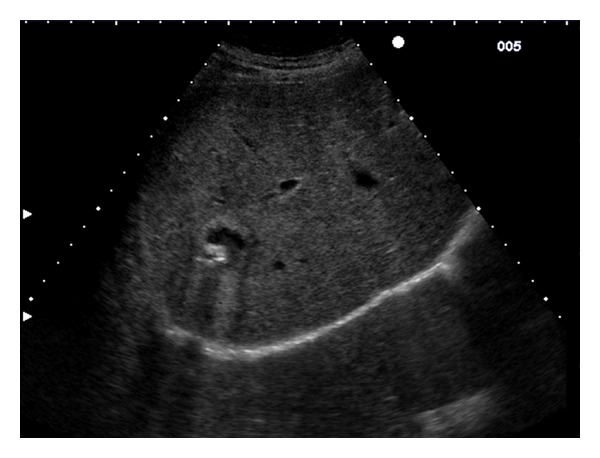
Ultrasound appearance of a brucellar abscess. A central dense calcium deposit is also observed with acoustic shadowing.
